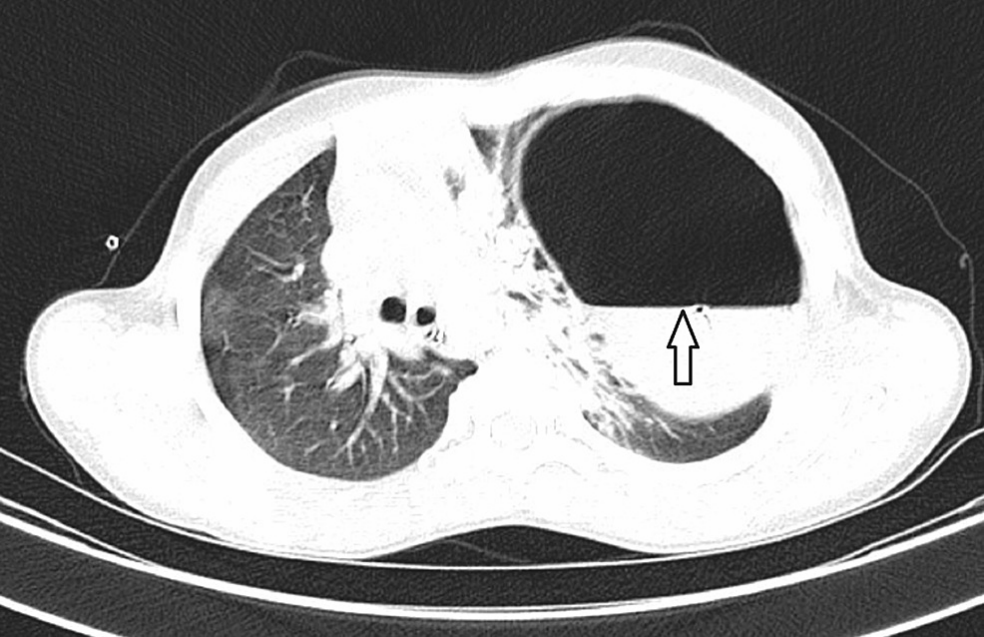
Cross-sectional CT view showing volvulus in herniated stomach
